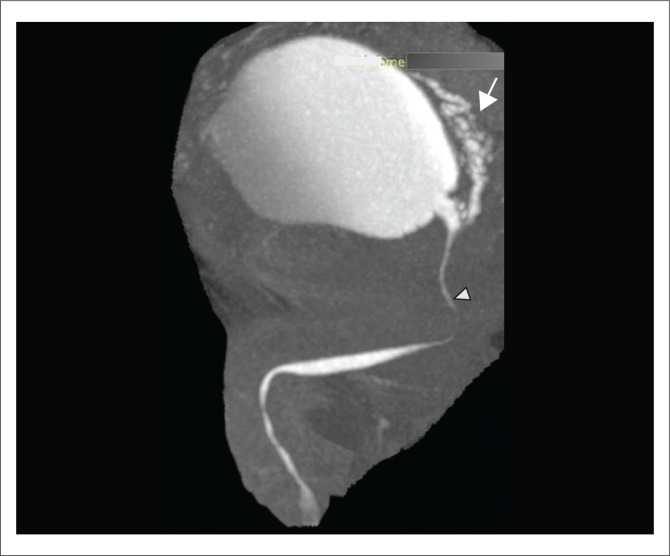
Sagittal maximum intensity projection (multidetector computed tomographic urethrography) showing reflux into the prostatic glands and seminal vesicles (arrow) and a urethral stricture of the entire posterior urethra. (arrowhead).
